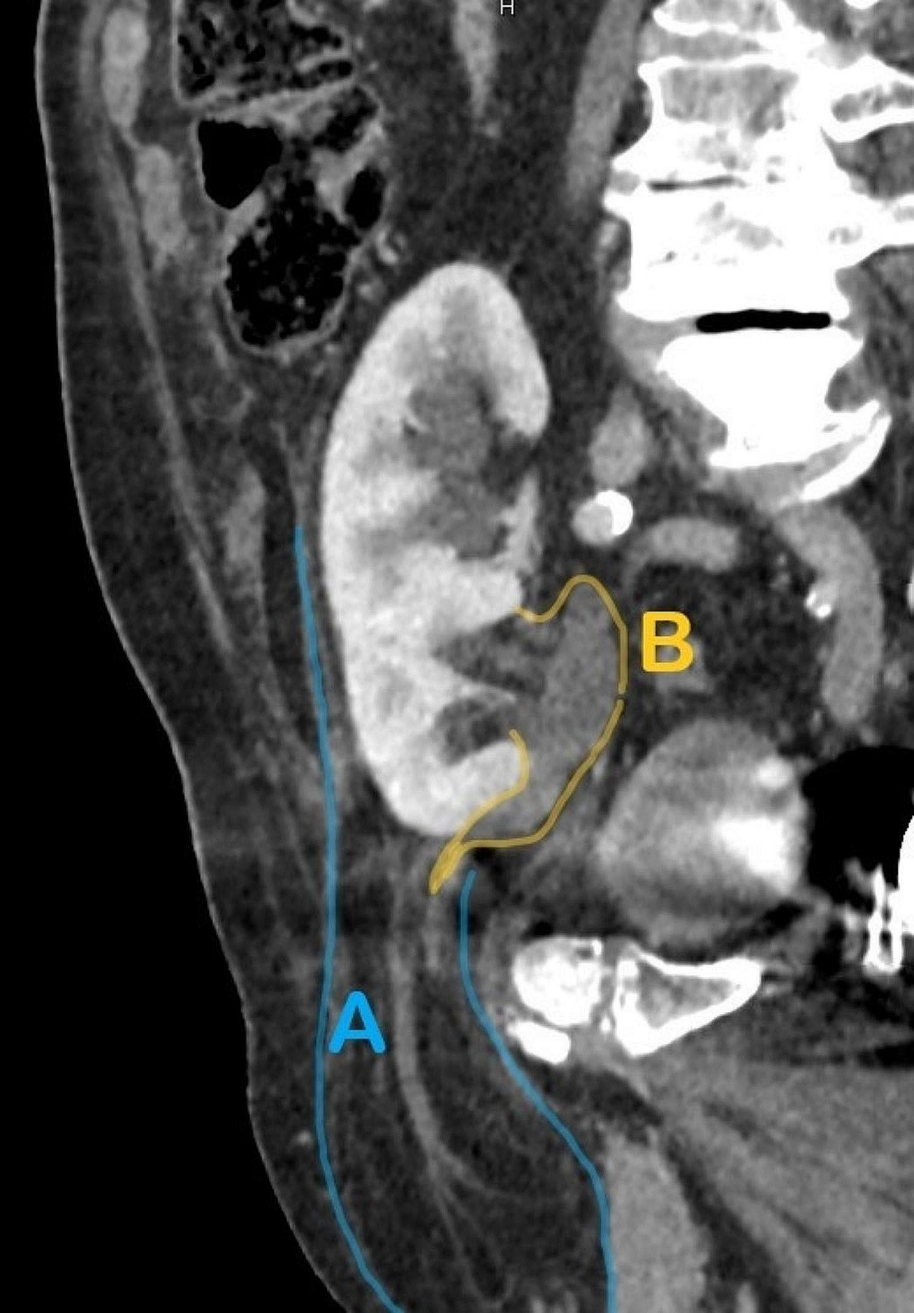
CT scan showing herniated perirenal fat (A) and dilated ureter with obstruction in the internal inguinal ring (B)
